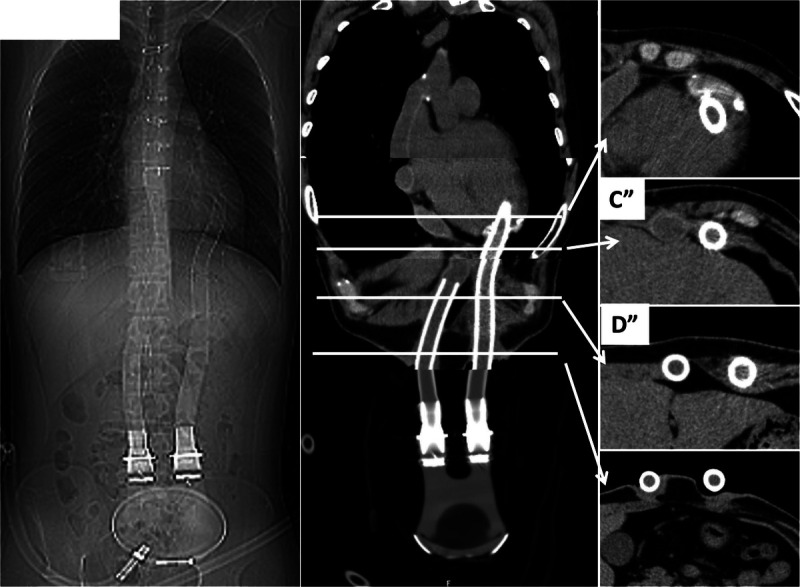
These accumulations correspond to the sites of insertion (CT scout obtained 14 years ago shows installed left ventricular assist device [LVAD] [left], reconstruction of CT coronal [middle], CT axial [right]; C″ and D″ correspond to the heights of C, C′, D, and D′ in Fig. 1, respectively) of inflow and outflow drive lines of an LVAD used for severe dilated cardiomyopathy before heart transplantation. Abnormal uptake is also seen in high-density structures in the right axillary artery, aortic arch, and left femoral artery on CT (Fig. 1: A, A′, B′, B, E, and E′), indicating accumulation in prostheses retained at the inflow insertion site during use of the extracorporeal circulation device. According to the International Society for Heart and Lung Transplantation registry, malignancy is the most common cause of death at 5 years after transplantation, accounting for more than 20%.^1^ The most common malignancies are skin cancers followed by posttransplant lymphoproliferative disorders (PTLDs).^1,2^ According to the systematic review and meta-analysis of Montes de Jesus et al, ^18^F-FDG PET/CT is currently the imaging modality most frequently used in PTLD patients and is useful for accurate diagnosis, staging, and biopsy site selection.^3^ Another meta-analysis reported high sensitivity and specificity of FDG PET for the diagnosis of PTLD (89.7% [95% confidence interval, 84.6–93.2] and 90.9% [85.9–94.3%], respectively),^4^ but false-positives due to factors such as infection and inflammation, including healing surgical scar, should be considered.^4–6^ FDG PET/CT is also a valuable tool for evaluating device-associated infections in patients with LVAD and has high sensitivity,^7^ but to the best of our knowledge, there are no reports of abnormal uptake at the site of insertion on ^18^F-FDG PET/CT after LVAD weaning and heart transplantation. In the present case, FDG uptake occurred at multiple subcutaneous sites. FDG uptake in surgical scars has been noted since the early days of FDG-PET^8^; however, persistent FDG uptake may be due to keloid formation or a foreign body response such as stitch granuloma.^9,10^
